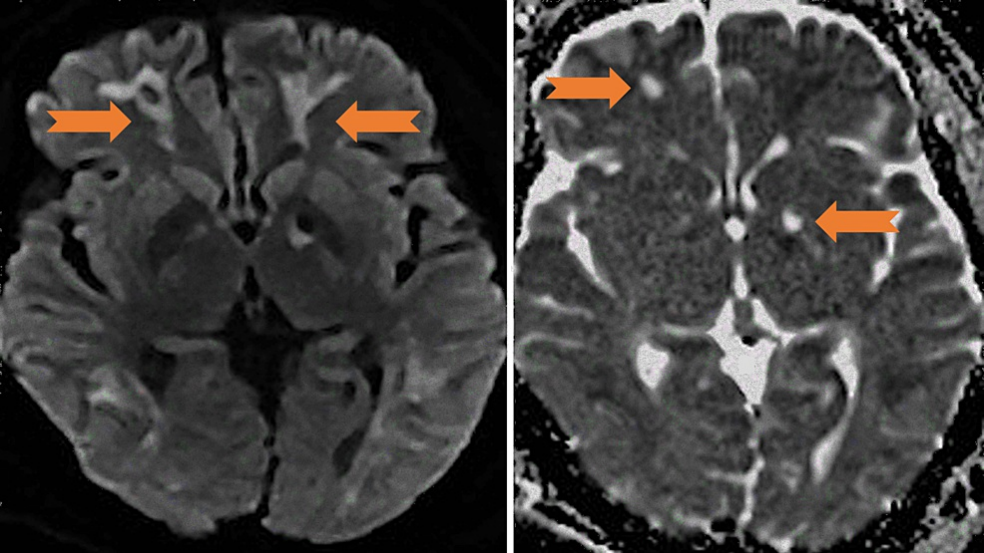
DWI (left) and ADC (right) with basal ganglia, left more than right diffusion restriction with ADC correlate (arrows). DWI: diffusion-weighted imaging; ADC: apparent diffusion coefficient
